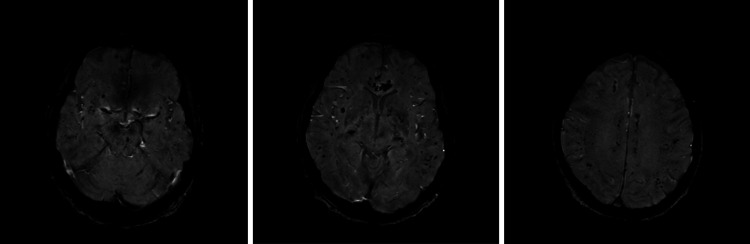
CT scan demonstrating the development of multiple small infarcts in a patient with respiratory and hematologic comorbidity factors.
